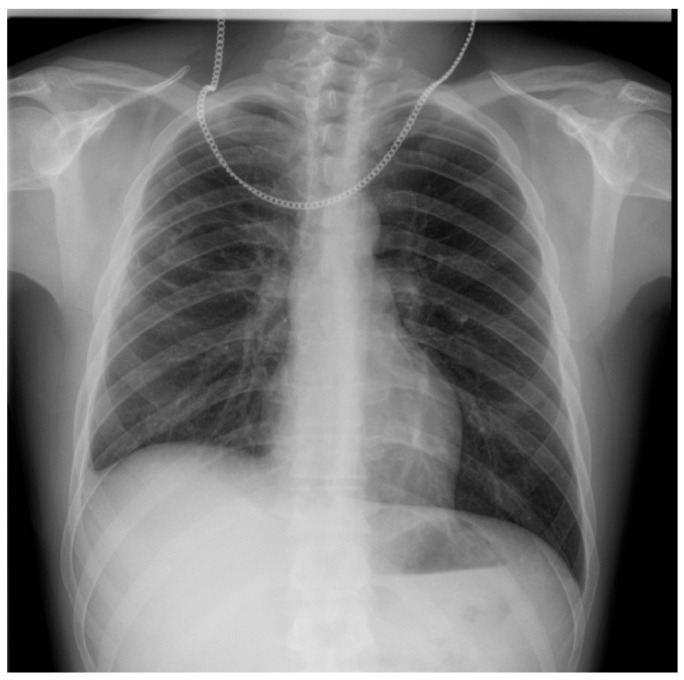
Chest-X-ray one-month after discharge from the Thoracic Surgery Department showing resolution of pleural fluid, complete lung re-expansion after surgical removal of large emphysematous bulla in the inferior right lobe.
